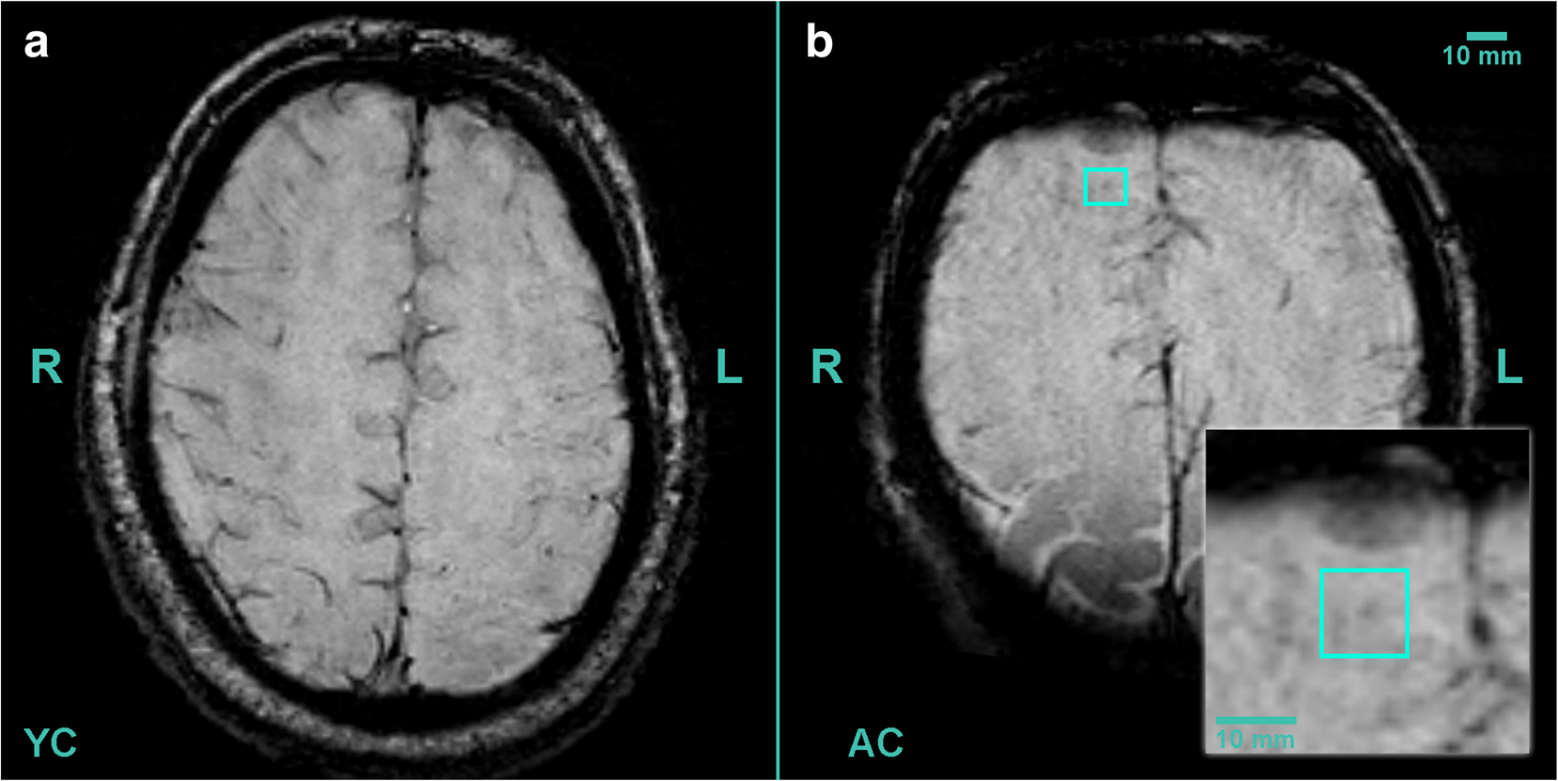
Axial (3 T) SWI MR images of aged and young control patients (without trauma). a Aged control (AC) patient presented one CMB lesion, located in right corona radiate, highlighted with blue square. b The young control (YC) patient had no cerebral lesion
